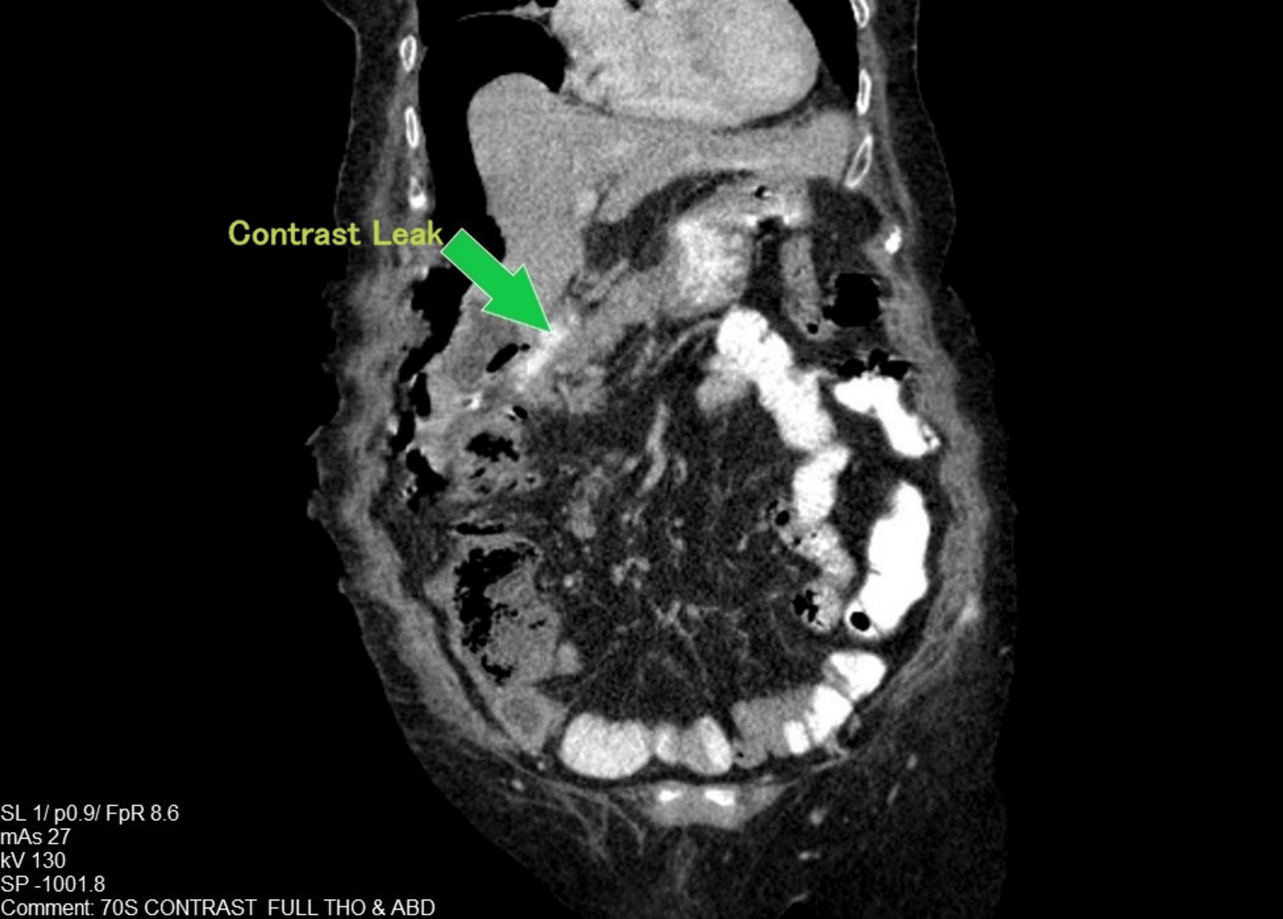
Coronal CECT demonstrating the site of duodenal perforation. Coronal view of a contrast-enhanced CT (CECT) scan showing oral contrast extravasation (contrast leak, marked by an arrow) from the second part of the duodenum. This radiological finding confirms the perforation and correlates with the free gas seen on the erect chest X-ray. Image credit: Department of Radiology, District General Hospital, Horana.
